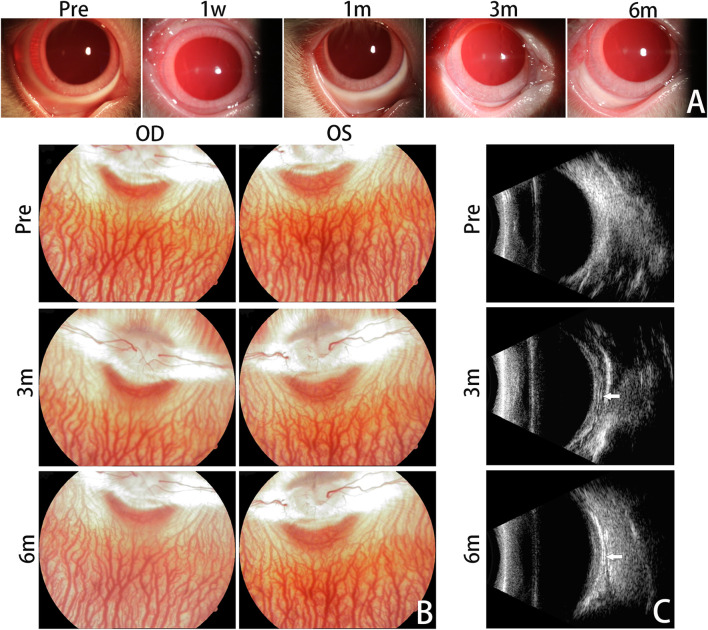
Clinical Evaluation. Anterior segment photographs are presented for the treated eyes before surgery and at 1 week, 1 month, 3 months, and 6 months after the surgery (A). No significant complications emerged within the 6-month follow-up period. Mild conjunctival congestion was observed at 1 week postoperatively and had completely resolved by the first month. Fundus photographs were taken to monitor the dynamic changes of the vitreous body and retina (B). No abnormal fundus changes were observed from 3 months to 6 months postoperatively, compared with pre-operation. Representative B-ultrasound images are displayed for the treated eyes before surgery and at 3 months and 6 months postoperatively (C). The silk fibroin graft could be clearly observed and was characterized by an arcuate band (white arrows). OD, oculus dexter; OS, oculus sinister.
